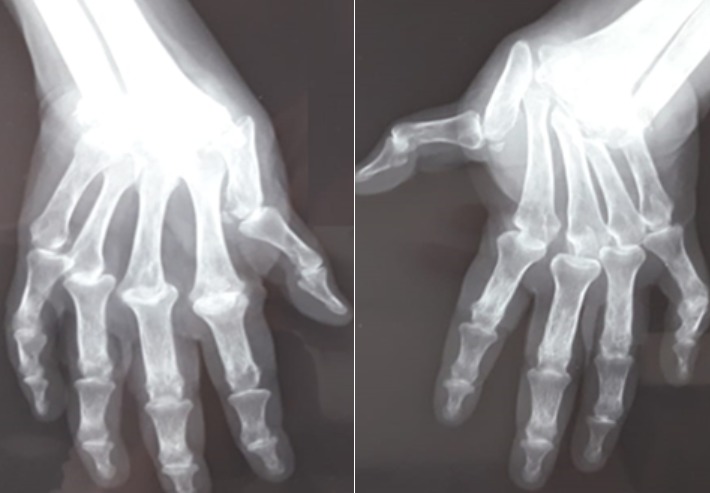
Standard radiograph of the hands of our case: 51-year-old woman with rheumatoid arthritis
